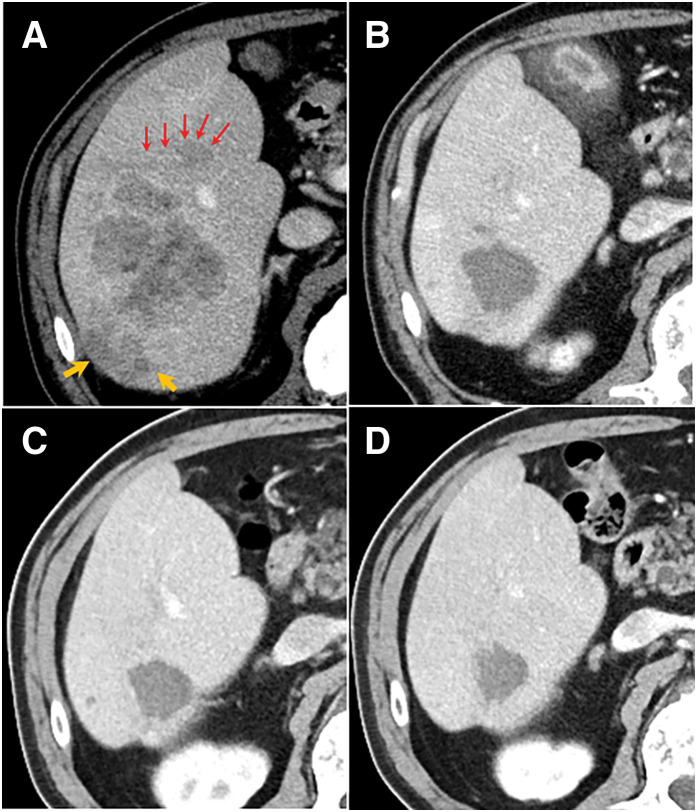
Radiological response of the primary hepatic tumor. (A) Pre-treatment contrast-enhanced abdominal CT showing a large, diffuse tumor (maximum diameter: 60 mm) in the posterior segment of the right hepatic lobe, with multiple daughter nodules (orange arrows) and invasion of the Glissonean branch of segment 6a (red arrows). (B) After three cycles of the STRIDE regimen, the hepatic tumor had shrunk significantly to 30 mm. (C) After six cycles, the primary tumor remained stable in size, and the daughter nodules became indistinct. (D) After nine cycles, the hepatic tumor had further shrunk to 25 mm, with complete disappearance of the daughter nodules.
